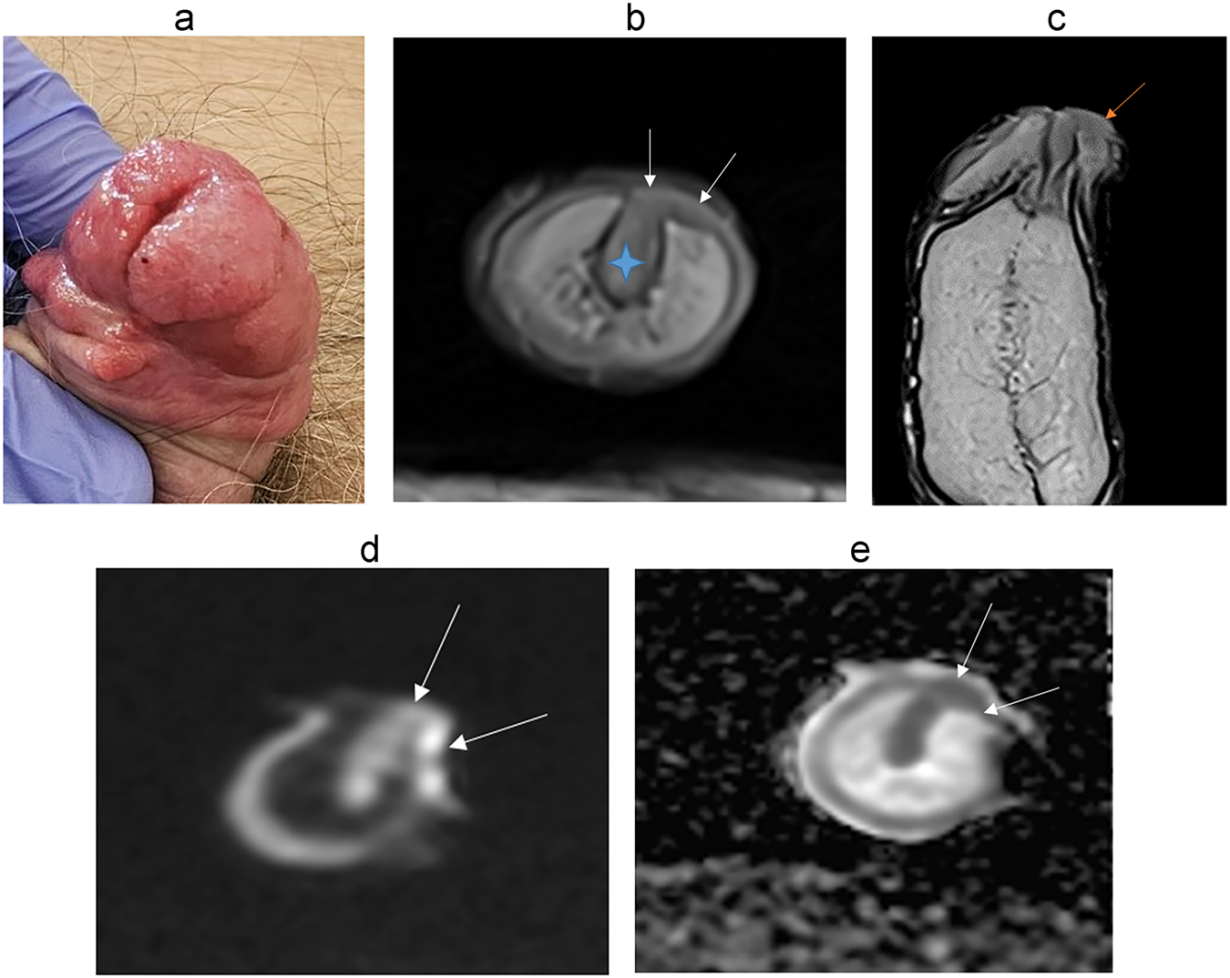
Ninety-one-year-old patient, referred for a cT1 lesion of the glans (a). b Axial, (c) coronal T2-W MR images show a superficial budding lesion of the glans predominant on the left ventral side (white arrows) with distal intra-urethral extension over approximately 8 mm (blue star). There is a millimetric focal invasion of the left corpus spongiosum of the glans on its distal ventral portion (orange arrow). d Axial DWI and (e) ADC map show intense diffusion restriction. Patient underwent partial distal amputation showing a moderately differentiated HPV-related squamous carcinoma with invasion of the distal penile urethra and infiltrating the corpus spongiosum. Stage pT2N0
